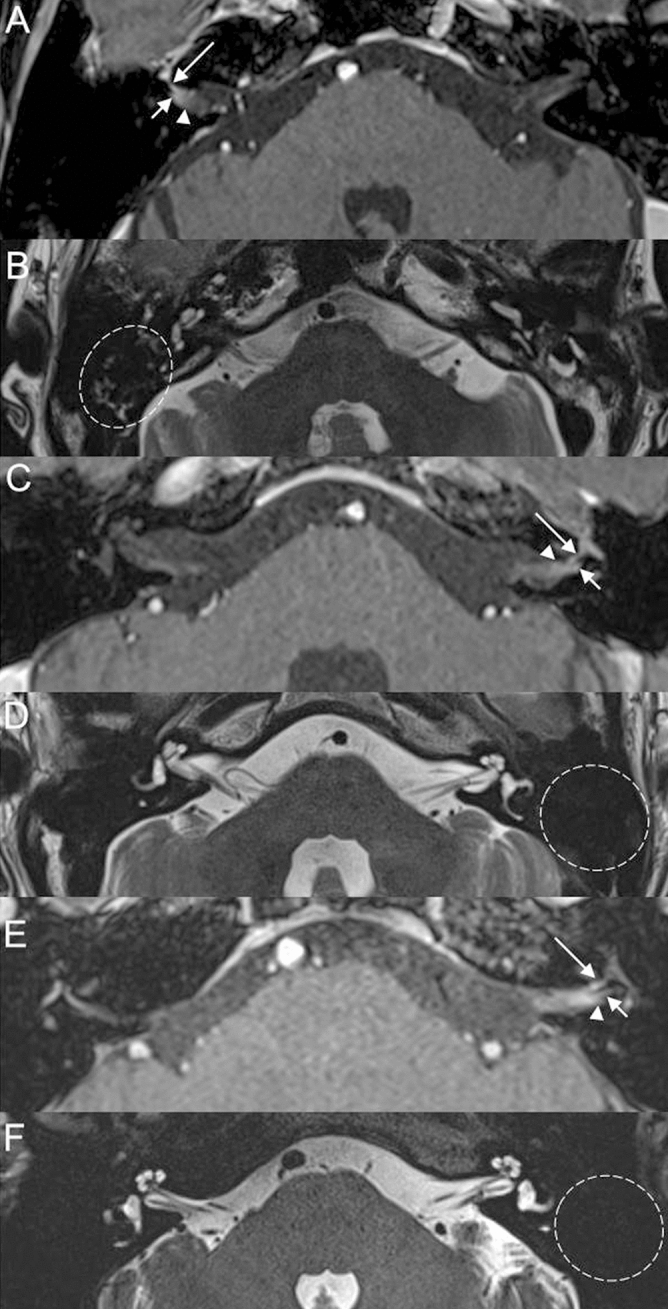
Temporal bone MRI of the patient with zoster sine herpete in the right side (A, B). (A) Post-contrast 3D T1 weighted image at the level of the internal auditory canal demonstrates enhancement of the labyrinthine segment of the right facial nerve (long arrow), right vestibular nerve (short arrow), and dura (arrowhead). (B) Non-contrast axial T2-weighted image shows high signal intensity from the fluid in the right mastoid suggesting mastoid effusion (dotted circle). Temporal bone MRI of the patient, who developed vesicular skin eruption in the left ear without acute facial nerve palsy (C, D). (C) Post-contrast 3D T1 weighted image at the level of the internal auditory canal demonstrates enhancement of the labyrinthine segment of the left facial nerve (long arrow), left vestibular nerve (short arrow), and dura (arrowhead). (D) Non-contrast axial T2-weighted image shows normal mastoid cavity on the left side (dotted circle). Temporal bone MRI of another patient, who developed vesicular skin eruption in the left ear without acute facial nerve palsy (E, F). (E) Post-contrast 3D T1 weighted image at the level of the internal auditory canal demonstrates enhancement of the labyrinthine segment of the left facial nerve (long arrow), left vestibular nerve (short arrow), and dura (arrowhead). (D) Non-contrast axial T2-weighted image shows normal mastoid cavity on the left side (dotted circle).
